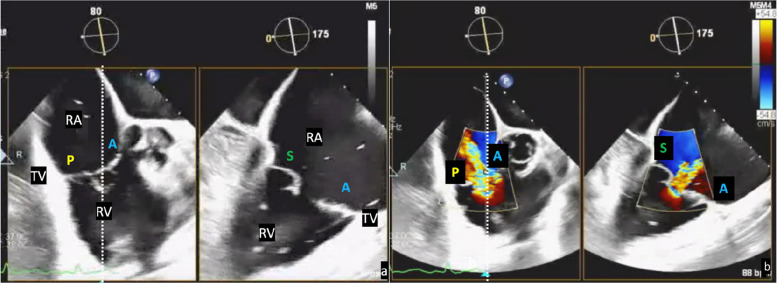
The mid-esophageal right ventricle (RV) inflow-outflow view. A Using simultaneous biplane imaging at RV inflow-outflow view (50°–70°), sweeping the orthogonal imaging plane (dotted line) from posterior (P; left side of primary view) to anterior (A; right side of primary view, near the aortic valve) visualizes the coaptation of the posterior-septal (P-S), and anterior-septal (A-S) commissures respectively. B The color flow Doppler from biplane imaging helps to localize the tricuspid regurgitation (TR) jet, which is more significant in central and anteroseptal aspect. RA, right atrium; RV, right ventricle; TV, tricuspid valve
